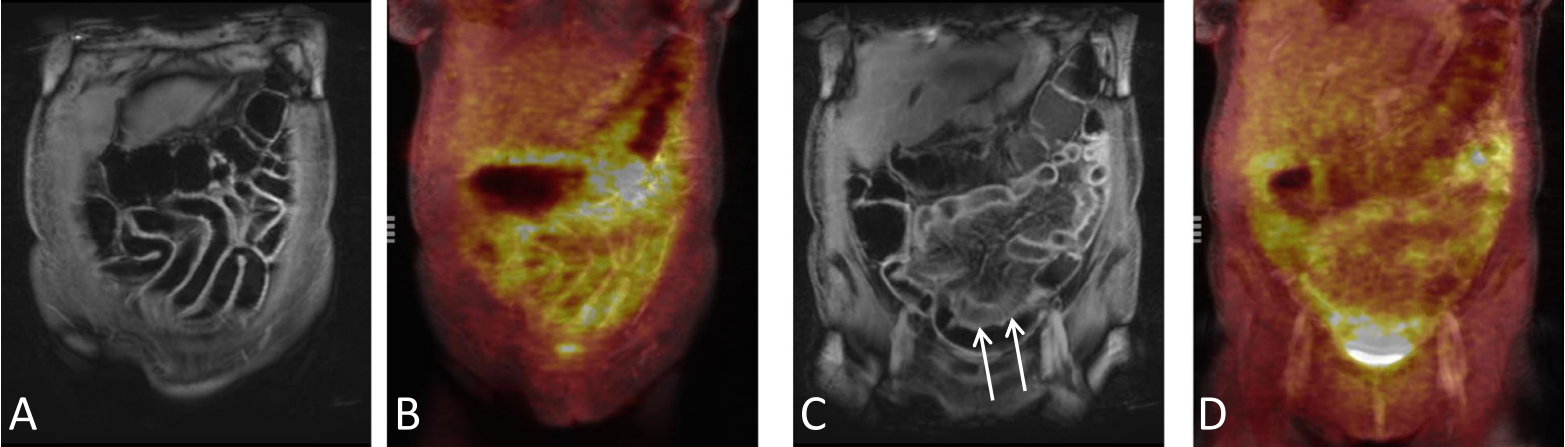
Coronal MR- and fused PET-MRI images of a patient with acute intestinal GvHD. Coronal T1-weighted MRI (A) reveals mural contrast enhancement of the small bowel loops with increased ^18^F-FDG uptake infused images (B). Typical hypervascular appearance of the mesentery (Comb sign) (arrows) adjacent to small bowel loops affected by GvHD (C) with increased ^18^F-FDG uptake in fused images (D)
